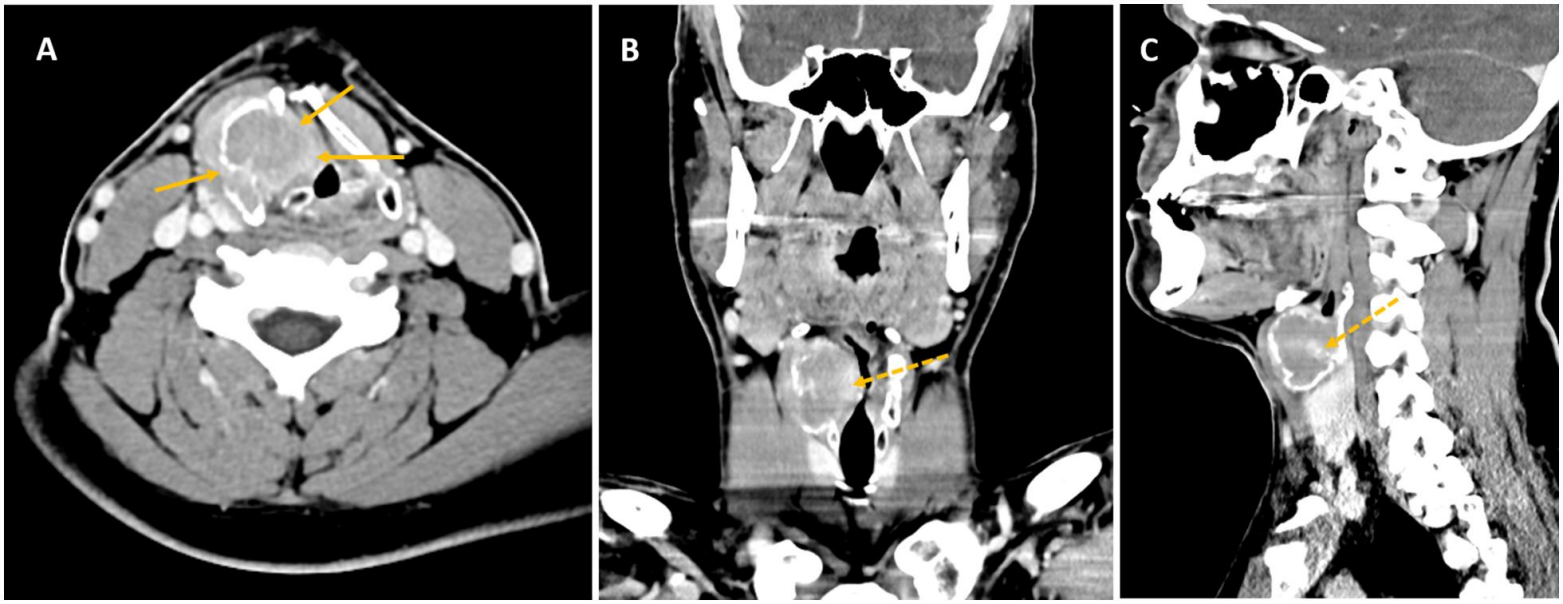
Computed tomography of the neck performed after intravenous iodine contrast administration on axial (A), coronal (B) and sagittal (C) planes show a large predominantly hypodense, lobulated mass expanding, and remodelling the right thyroid lamina with areas of cortical dehiscence more prominent in the inner cortex (solid arrows on A). The mass bulges into the paraglottic space and reduces the caliber of the glottic and supraglottic airway, which is displaced to the left. Cranio-caudally it extends from the inferior margin of the hyoid bone to the vocal process of the arytenoid cartilages. Note the presence of peripheral contrast enhancing soft tissue components (dashed arrows on B and C) and the absence of internal osteoid matrix or chondroid calcifications.
